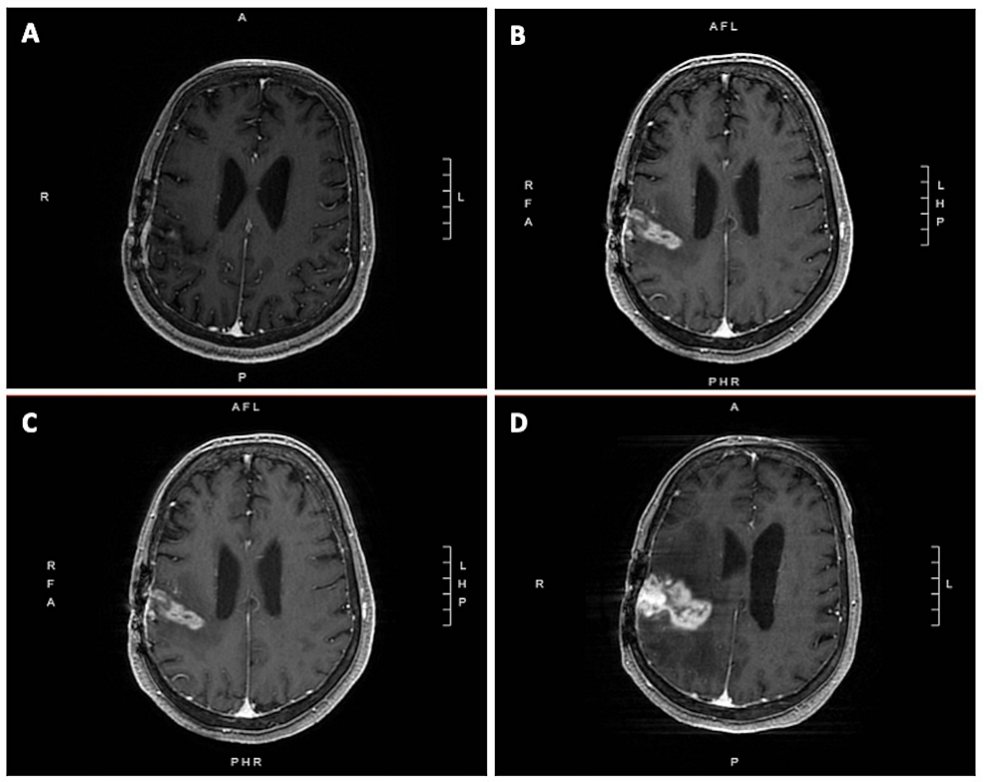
Surveillance imaging Serial T1 MRI with contrast reveals the gradual worsening of radiation necrosis at the treated resection cavity from approximately (A) three months, (B) six months, (C) 11 months, and (D) 13 months after completion of cavity SRS. SRS: stereotactic radiosurgery
